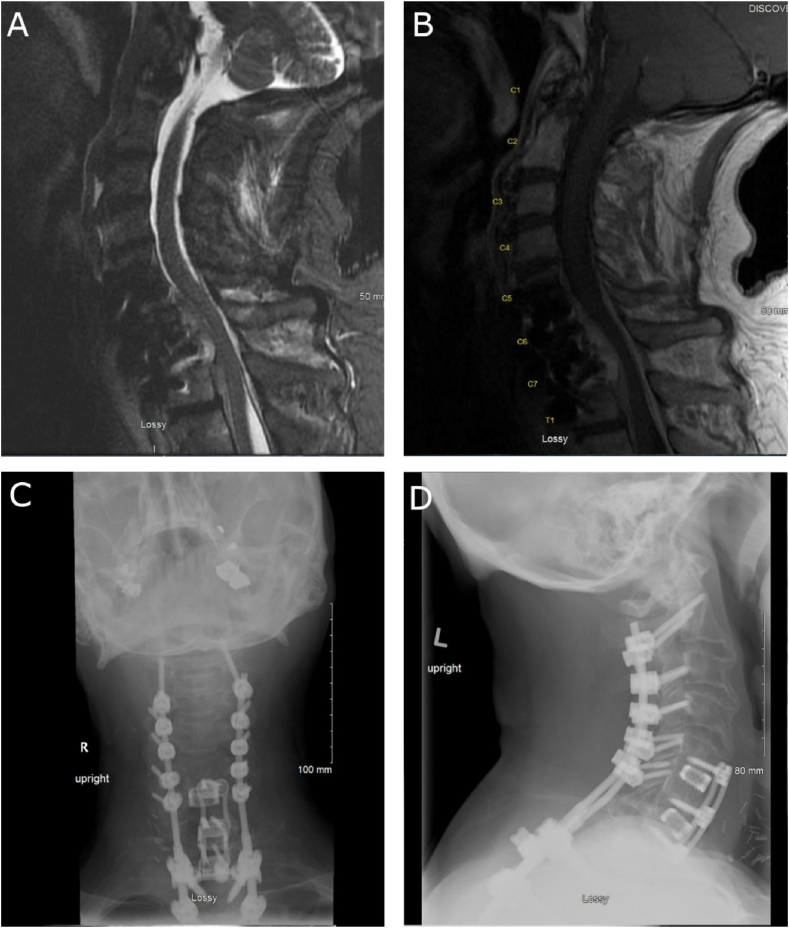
Example of a case of cervical spondylodiscitis with epidural abscess treated at our center. A: Sagittal STIR image demonstrates significant compression of the lower cervical spinal cord at the levels of C6-T1. Subtle increased intensity in the cord at this level. B: Sagittal T1 post-contrast image demonstrates significant contrast enhancement within the epidural space ventrally at the levels of C5-T1 as well as dorsally from C5-T2. There is also evidence of extension of the infection into the C5–C7 prior fusion. Focal kyphosis can also be seen at the level of C7/T1. C–D: Post-operative anterior-posterior (C) and lateral (D) plain x-ray films demonstrating posterior instrumentation from C2-T4 as well as stable anterior C5-7 prior instrumentation. Spinal alignment is improved compared to pre-op.
